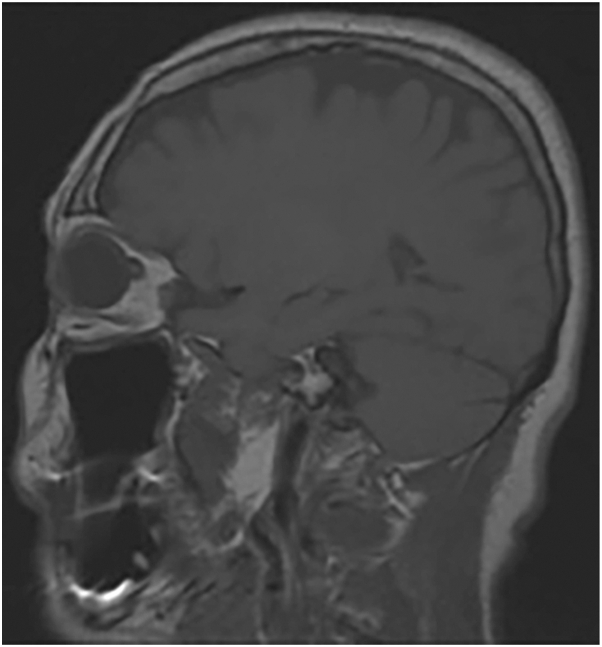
Sagittal view of the brain MRI demonstrating thinning of the right frontal calvarium. *MRI*, Magnetic resonance imaging.
